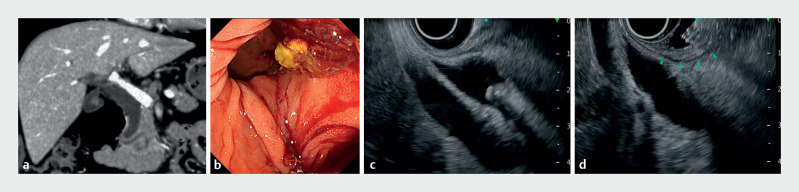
Gel immersion technique with the endoscopic ultrasound-guided rendezvous (EUS-RV) method for a difficult biliary cannulation case.
